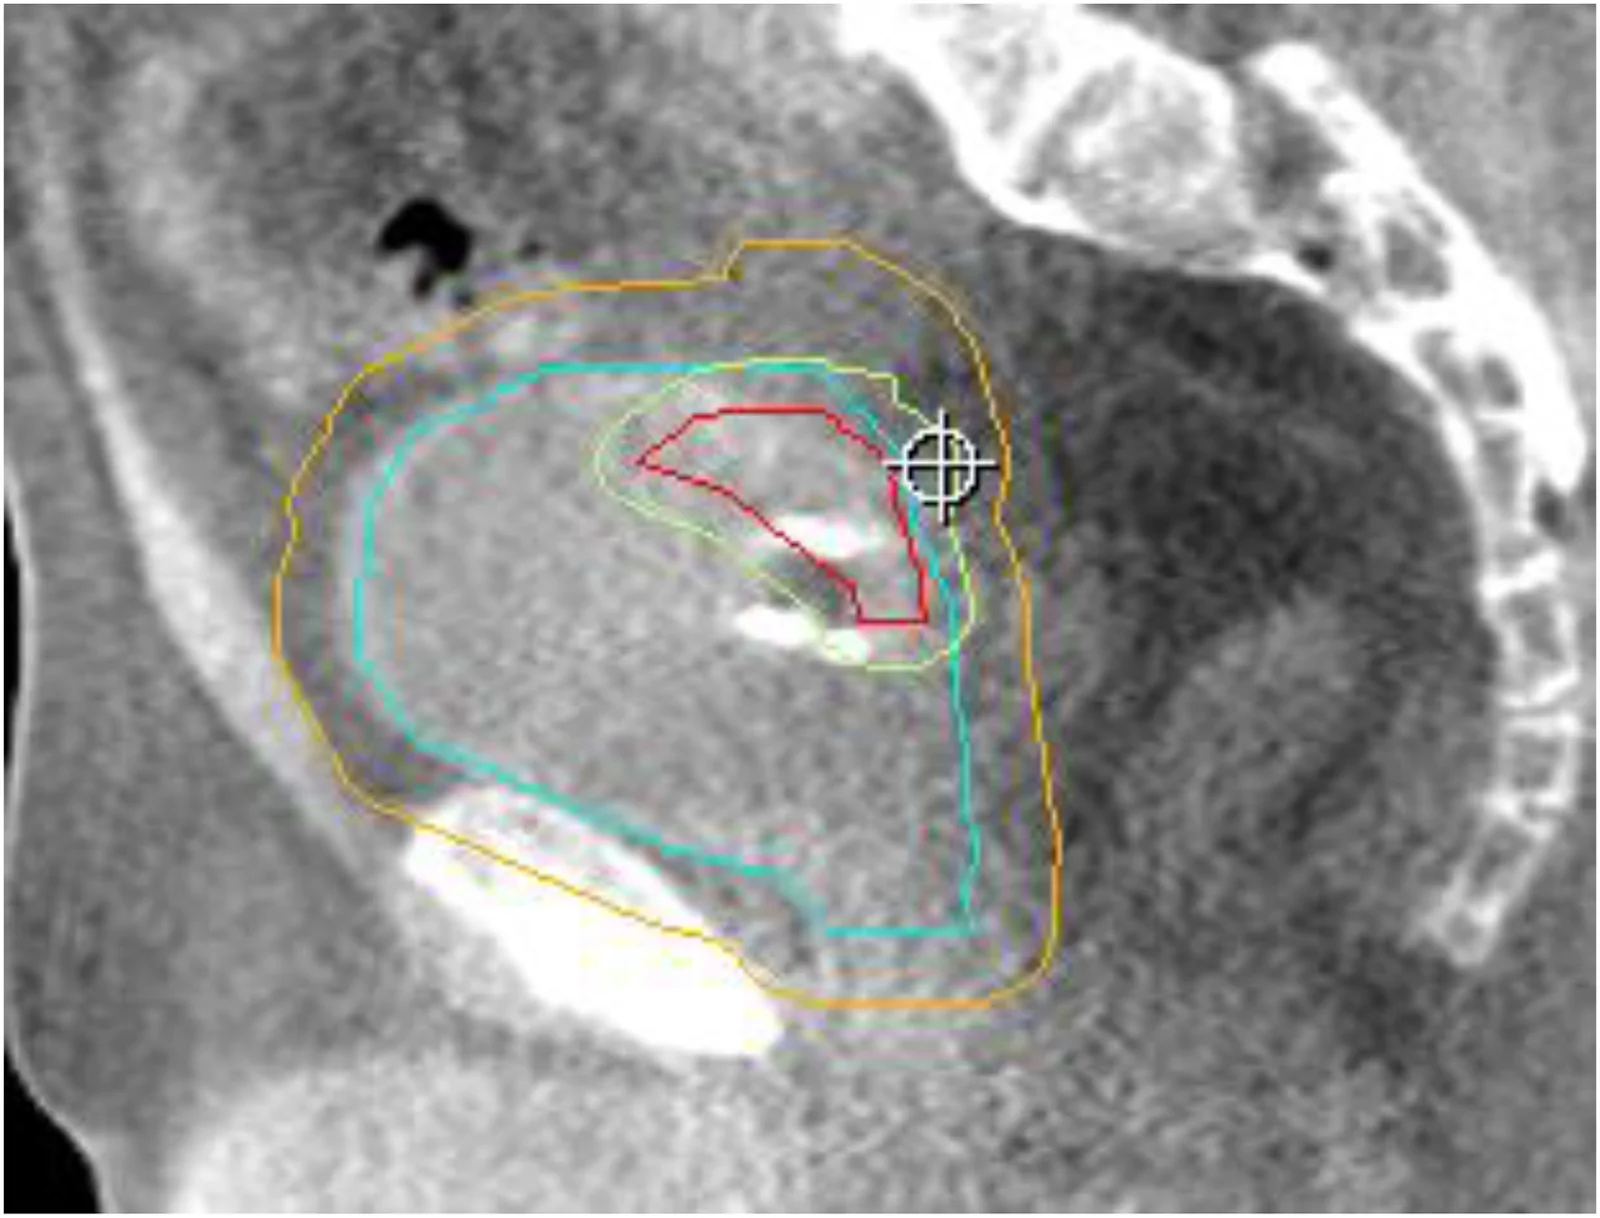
CBCT demonstrating the bladder is within the PTV. CTV whole bladder (teal), PTV whole bladder (orange), GTV boost (red), PTV boost (green). *Abbreviations:* CBCT = cone beam CT; CT = computed tomography; CTV = clinical target volume; GTV = gross target volume; PTV = planning target volume.
